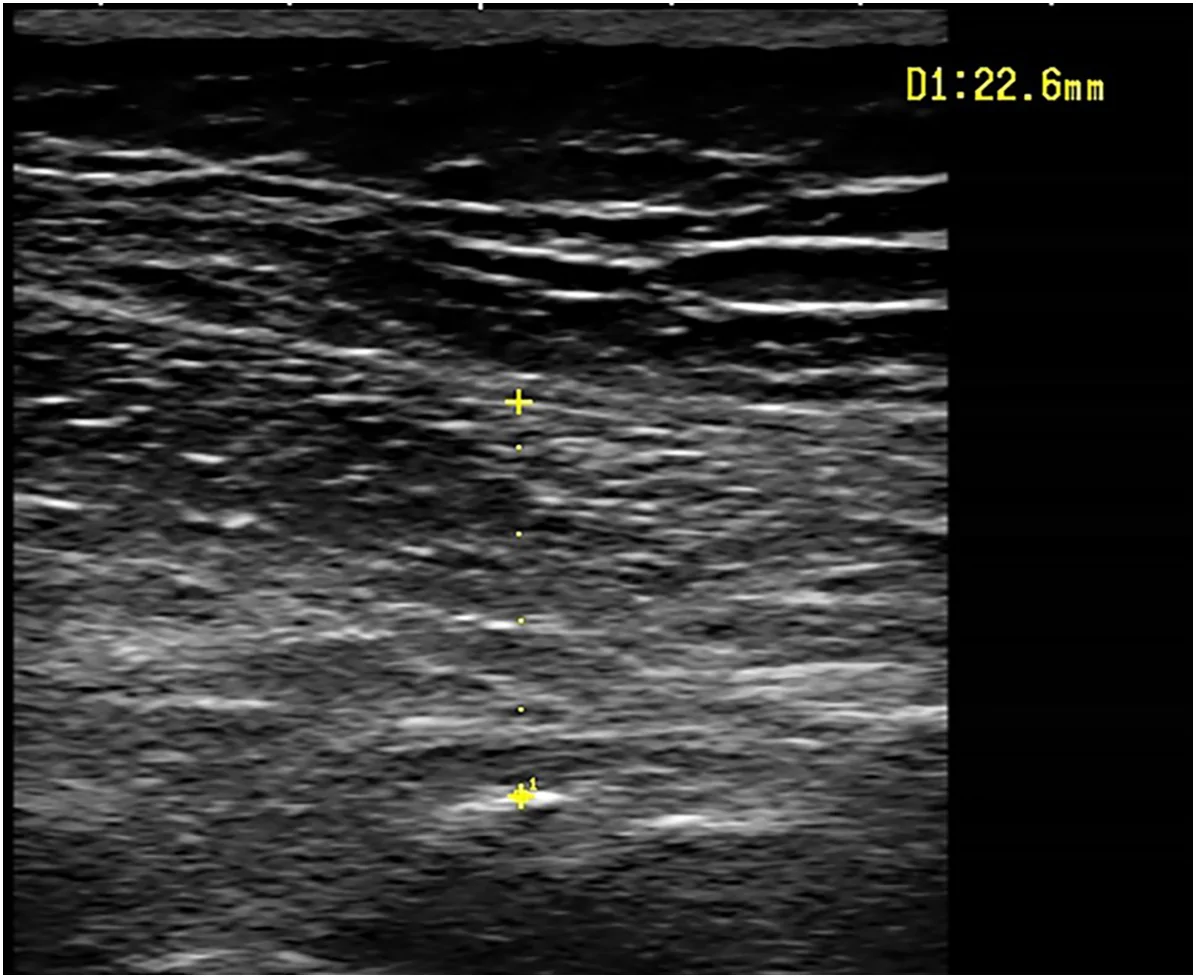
Ultrasound image of the thickness of the Multifidus muscle during contraction. Created by the authors. Published under CC BY 4.0.
